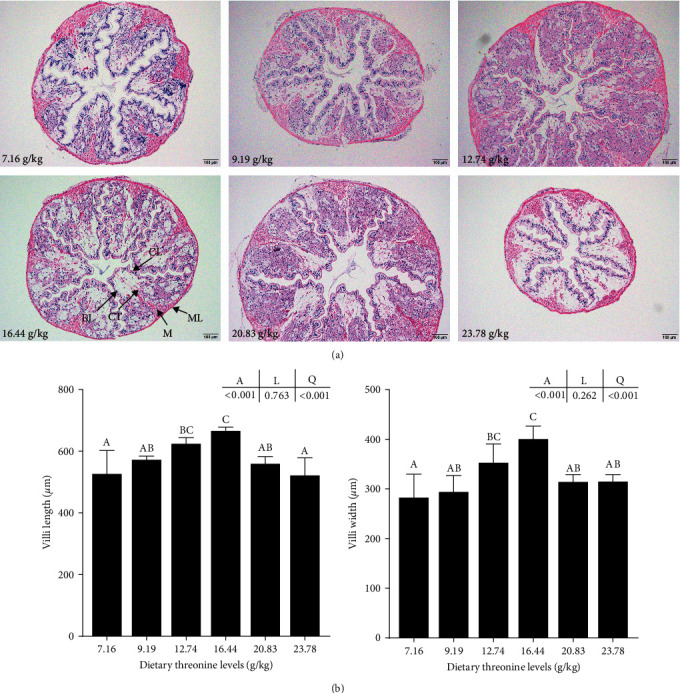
Effect of dietary threonine levels (dry matter basis, g/kg) on the histomorphology of intestinal of red swamp crayfish. (a) CL, chitin layer; EL, epithelium; CT, connective tissue layer; M, muscle bundle; and ML, muscle layer. The magnification is 100x. Scale bar = 100 *μ*m. (b) Effects of dietary threonine levels on the length and width of intestinal villi of red swamp crayfish. Data were expressed as mean ± SD (*n* = 3). Different letters above the vertical bars represent significant differences (*P* < 0.05). A, *p*-value of the variance analyzed by one-way ANOVA; L, *p*-value of linear trend analyzed by orthogonal polynomial contrasts; and Q, *p*-value of quadratic trend analyzed by orthogonal polynomial contrasts.
